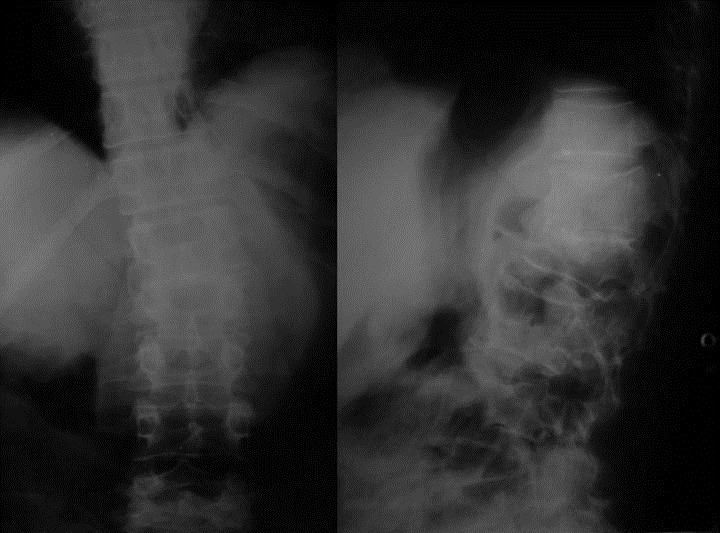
Anteroposterior and lateral radiograph of lumbar spine of same patient showing Pott's spine involving L1 and L2
